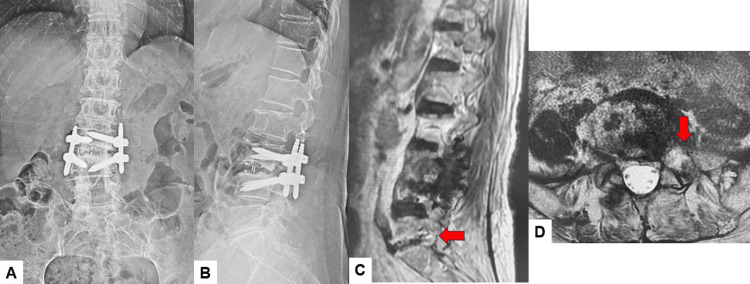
Postoperative radiographs and MRI. A: Anteroposterior lumbar radiograph; B: lateral lumbar radiograph; C: left parasagittal T2-weighted image; D: axial T2-weighted image at the L5/S1 level. Red arrows indicate adequate nerve decompression.
